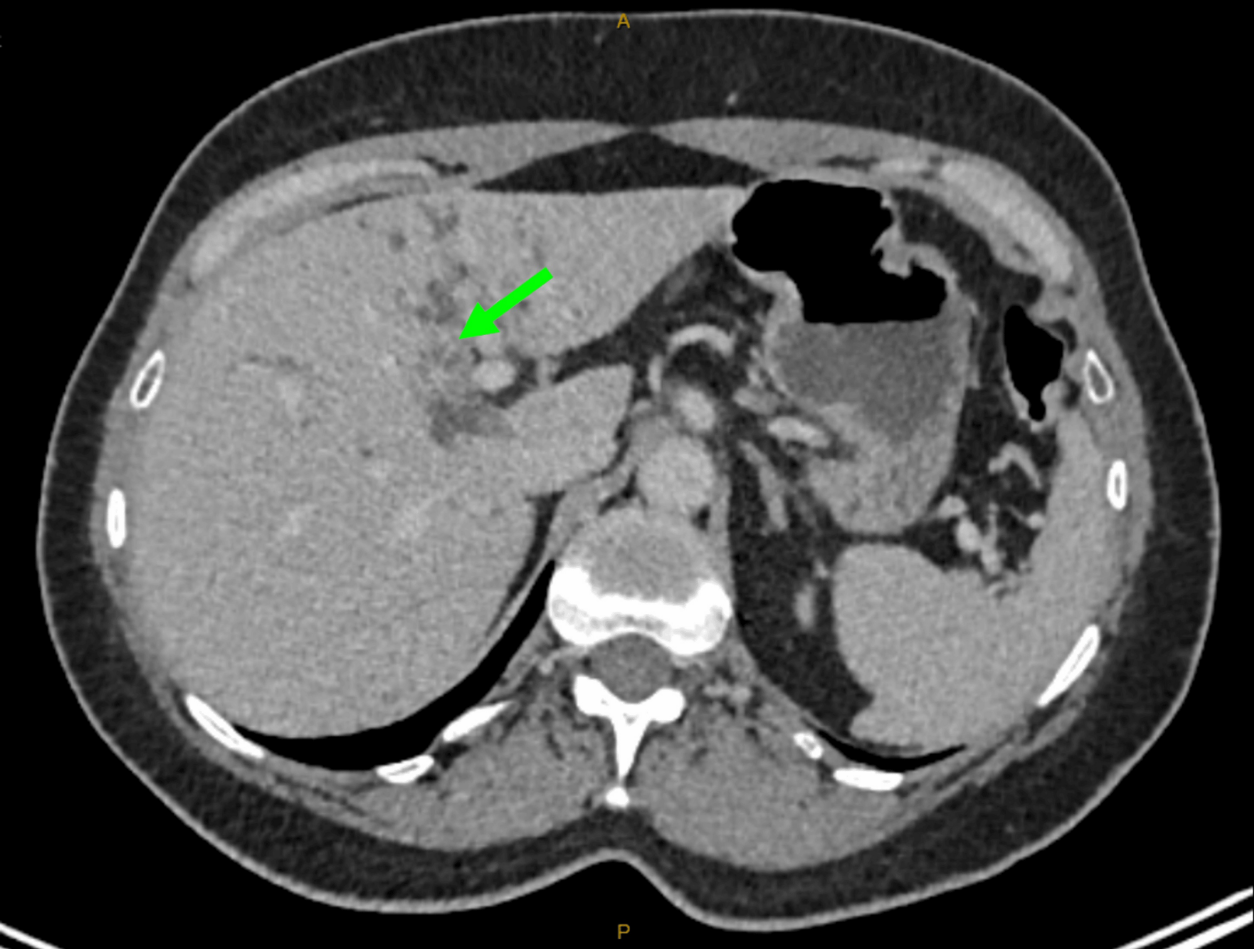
CT findings are highly suspicious for cholangiocarcinoma centered on the left lobe of the liver Green arrow: Tumor at the porta hepatis
